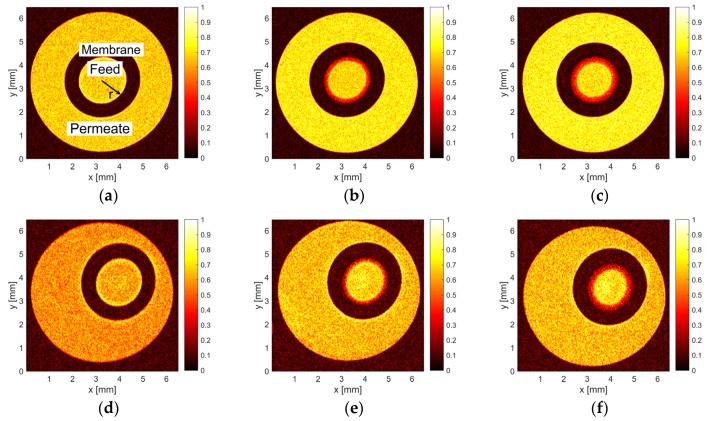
Axial MR images were measured every 5 min at *z* = 29 cm during dead-end filtration at *p* = 1.25 bar and different temperatures *ϑ* = 15 °C (a–c) and *ϑ* = 45 °C (d–f). The normalised MR intensity *I*/*I*_max_ is indicated in the false-colour bar. (a,d) A reference image of the unused hollow fibre was acquired before each filtration. (b) Build-up of the protein deposit at *t* = 79 min of filtration (red ring at the inner surface of the membranes lumen) and *t* = 151 min (c) for the lowest temperature *ϑ* = 15 °C. Progressing accumulation of the deposit was observed on the selective layer of the hollow fibre membrane at *t* = 67 min (e) and 168 min (f) during the dead-end filtration at *ϑ* = 45 °C.
